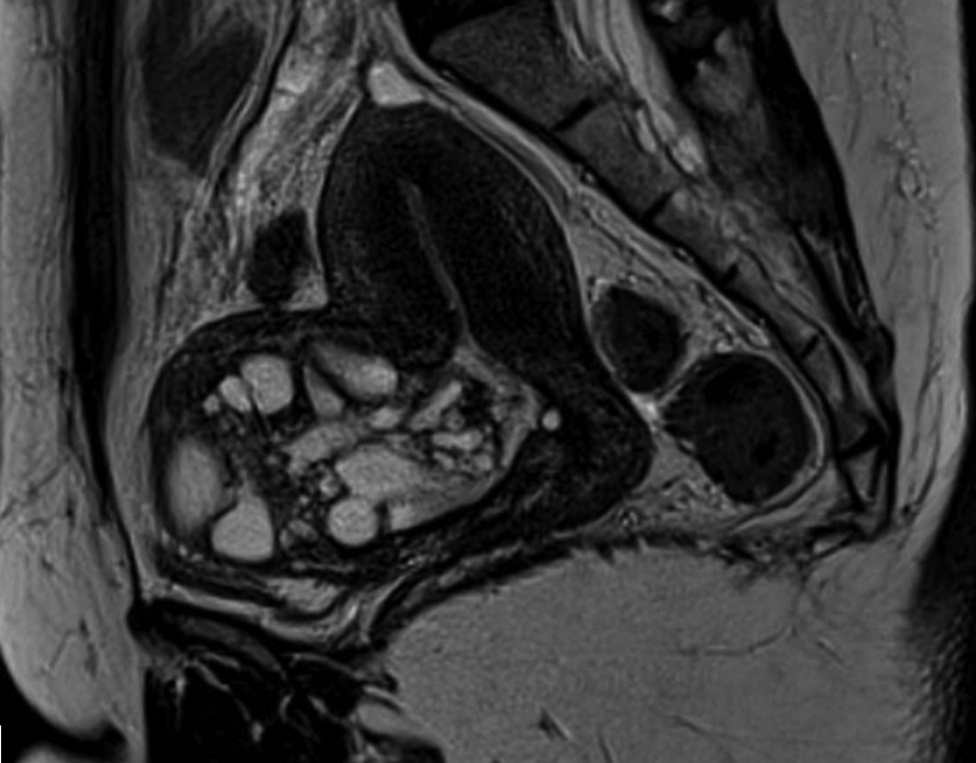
Magnetic Resonance Imaging (MRI) demonstrating a bulky isthmocele at cesarean section scar with several cysts inside.
